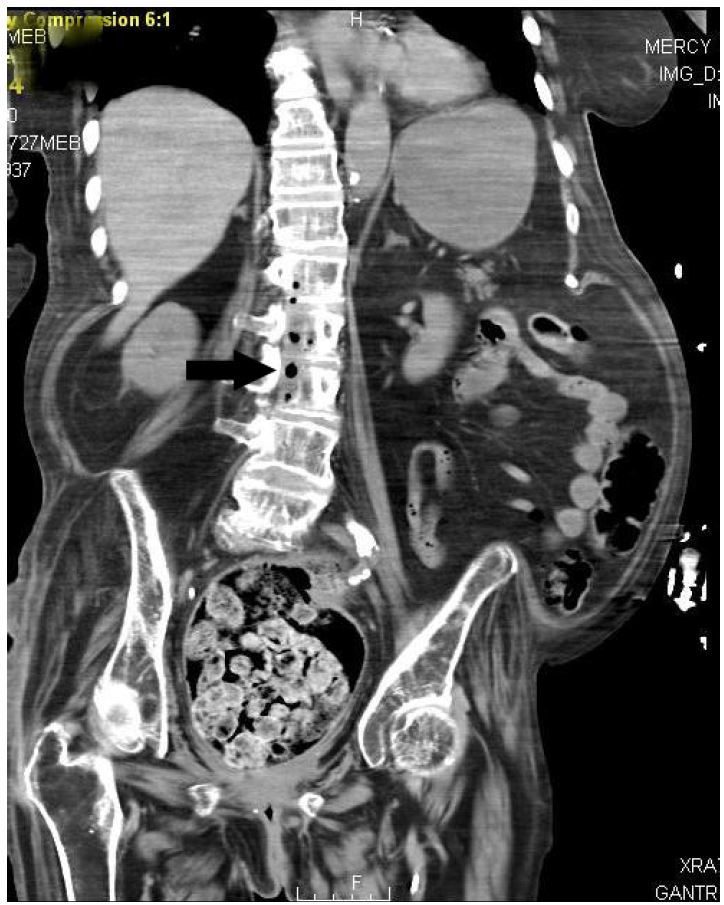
Computed tomography reconstructed coronal image of the abdomen and pelvis demonstrating gas in the thoracolumbar spinal canal (arrow).
